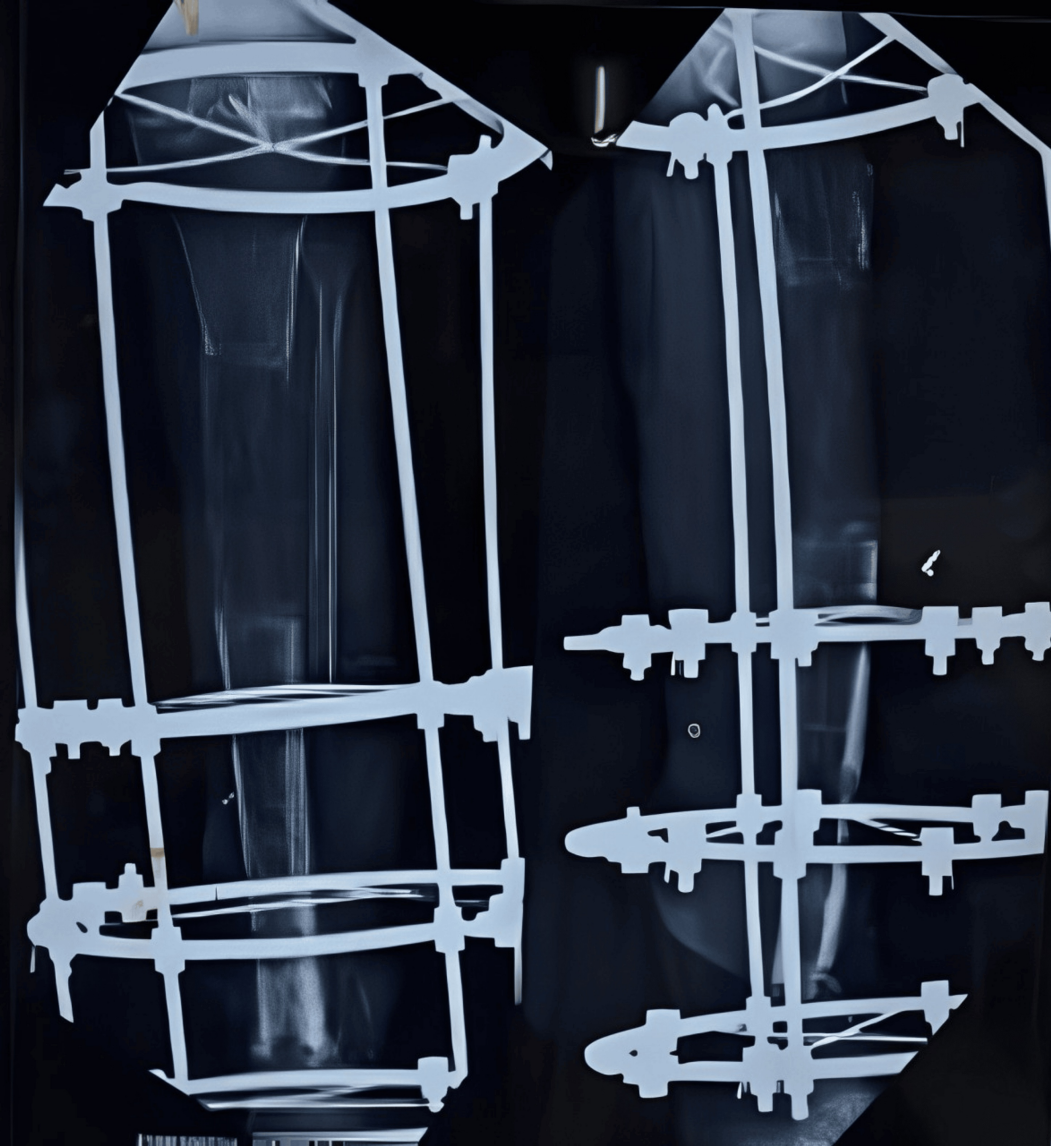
X-ray showing the completed distraction (at the end of six months)
